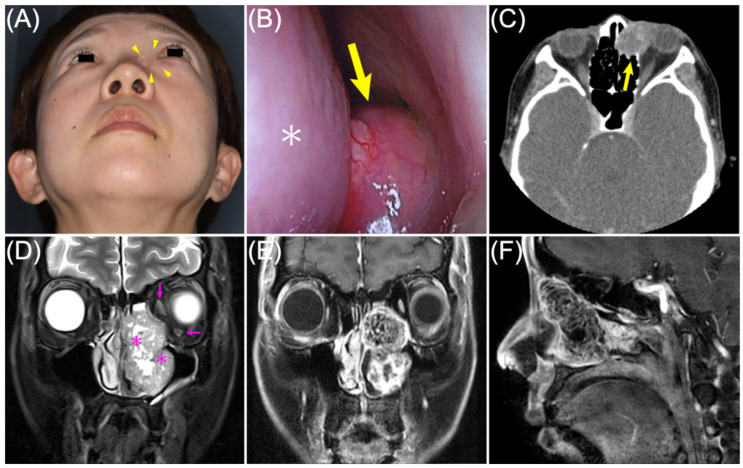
Preoperative clinical, endoscopic, and radiologic findings suggesting a large lacrimal drainage system tumor. (A) Upward-view facial photograph showing left-sided proptosis and a firm mass in the left medial canthal/lacrimal sac region, indicated by yellow arrowheads; (B) Nasal endoscopy showing an intranasal mass (yellow arrow) adjacent to the middle turbinate (white asterisk); (C) Contrast-enhanced computed tomography at the lacrimal sac level showing a mass centered in the left lacrimal sac region (yellow arrow); (D) Coronal T2-weighted magnetic resonance imaging showing a large mass extending from the lacrimal sac region into the nasal cavity and maxillary sinus. The extraocular muscles are displaced (magenta arrows), and flow voids are visible within the tumor (magenta asterisks); (E) Coronal gadolinium-enhanced T1-weighted magnetic resonance imaging showing heterogeneous enhancement of the lesion; (F) Sagittal gadolinium-enhanced T1-weighted magnetic resonance imaging showing the craniocaudal and anteroposterior extent of the lesion.
